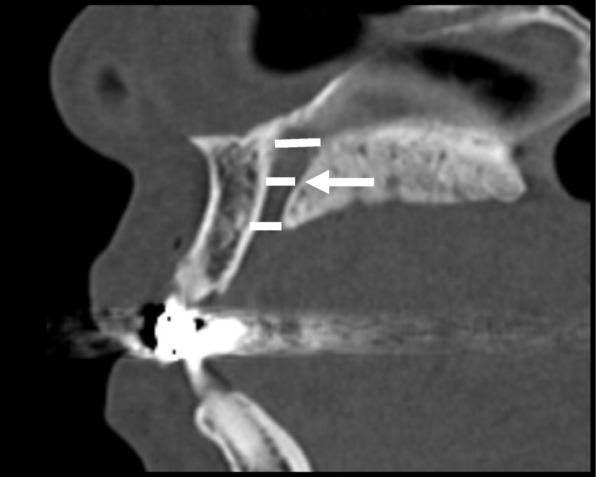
Based on CT sagittal sections of the incisive canals, the diameters of the nasal foramina are marked at the nasal entrances of the maxillary incisive canals, and the diameters of the incisive foramina are marked at the oral entrances of the canals. The canal diameters are measured at the midpoints between these two levels
